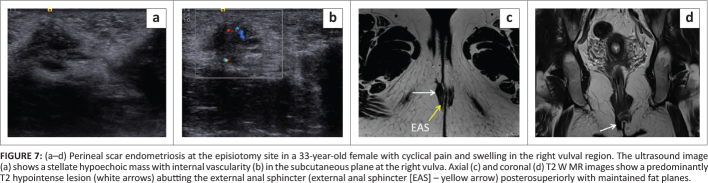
(a–d) Perineal scar endometriosis at the episiotomy site in a 33-year-old female with cyclical pain and swelling in the right vulval region. The ultrasound image (a) shows a stellate hypoechoic mass with internal vascularity (b) in the subcutaneous plane at the right vulva. Axial (c) and coronal (d) T2 W MR images show a predominantly T2 hypointense lesion (white arrows) abutting the external anal sphincter (external anal sphincter [EAS] – yellow arrow) posterosuperiorly with maintained fat planes.
